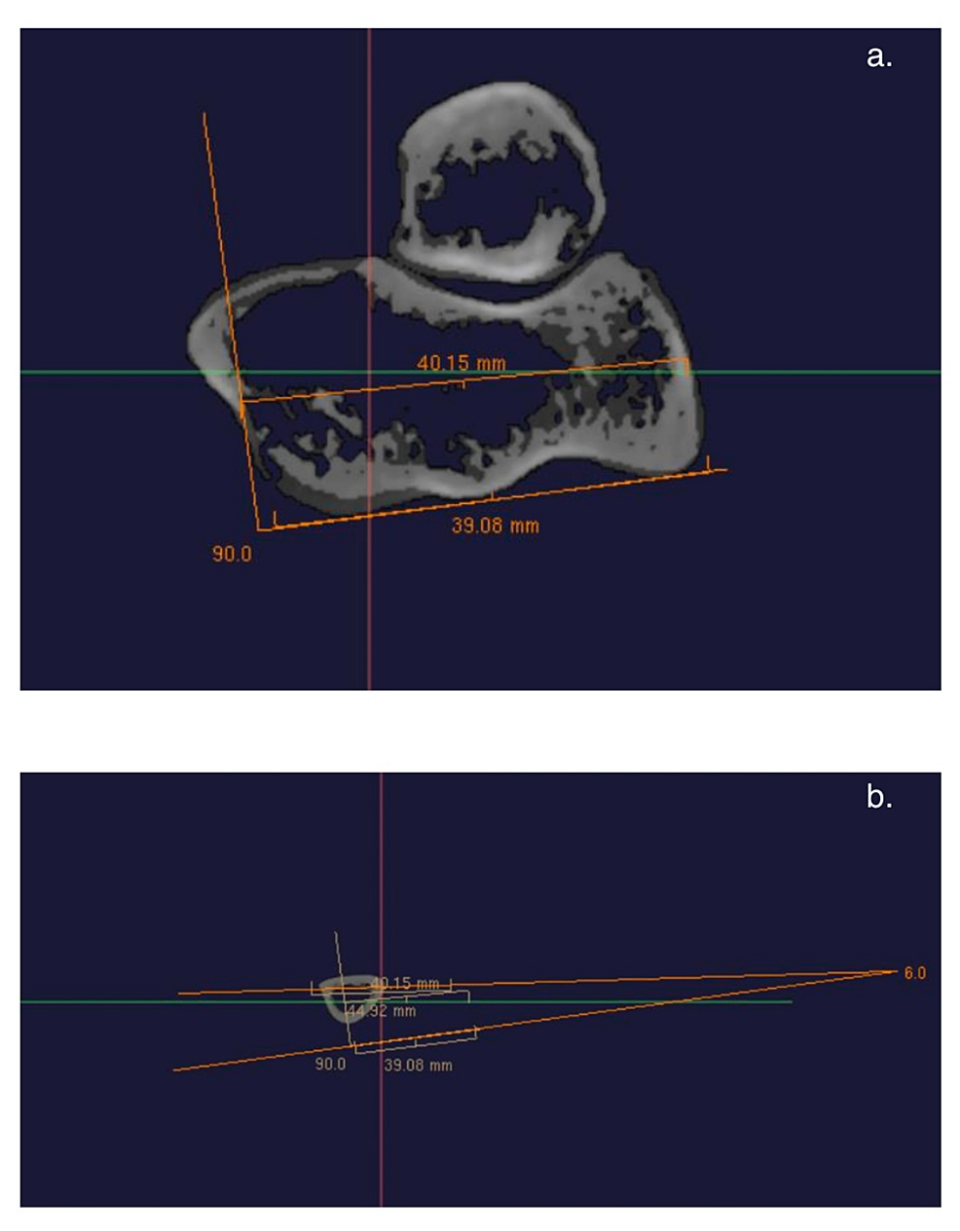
CT measurements of the intermuscular septum. Measurements of the intermuscular septum axis drawn proximal to the olecranon fossa (a) and viewed in the axial plane (b).
